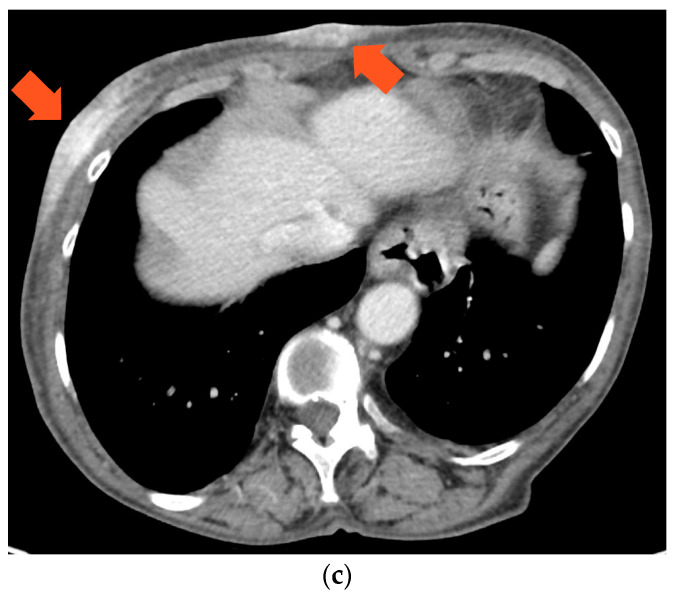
(a,b) Chest wall recurrence and (c) tumor cutaneous infiltration at the CT scan.
